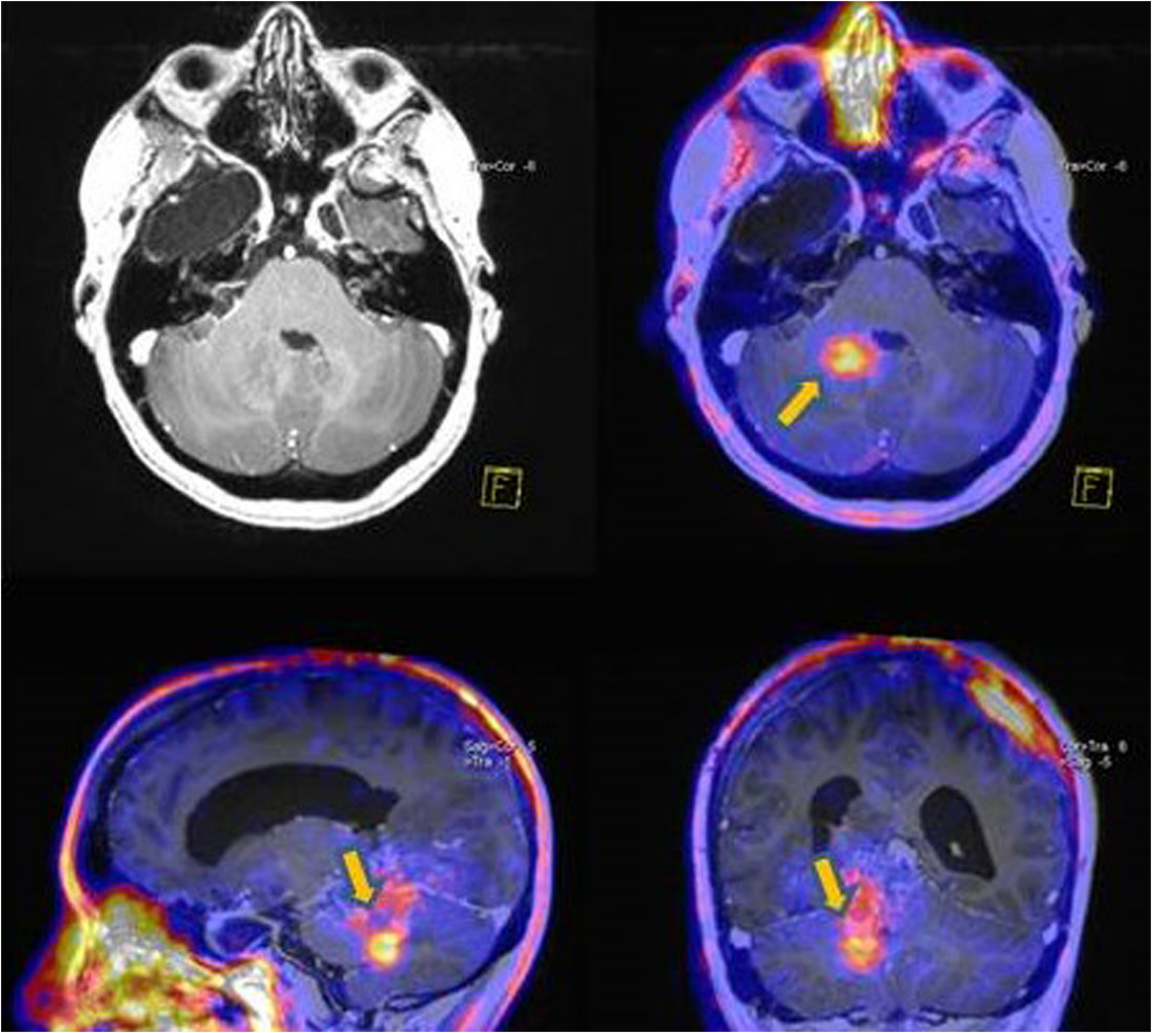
Anaplastic oligoastrocytoma grade III recurrence. Increased uptake of FLT at the right cerebral hemisphere (SUV_max_ 1.22, tumor-to-background ratio 4.8). Fusion of PET and MRI images. FLT* fluorothymidine, *MRI* magnetic resonance imaging, *PET* positron emission tomography, *SUV* standardized uptake value
